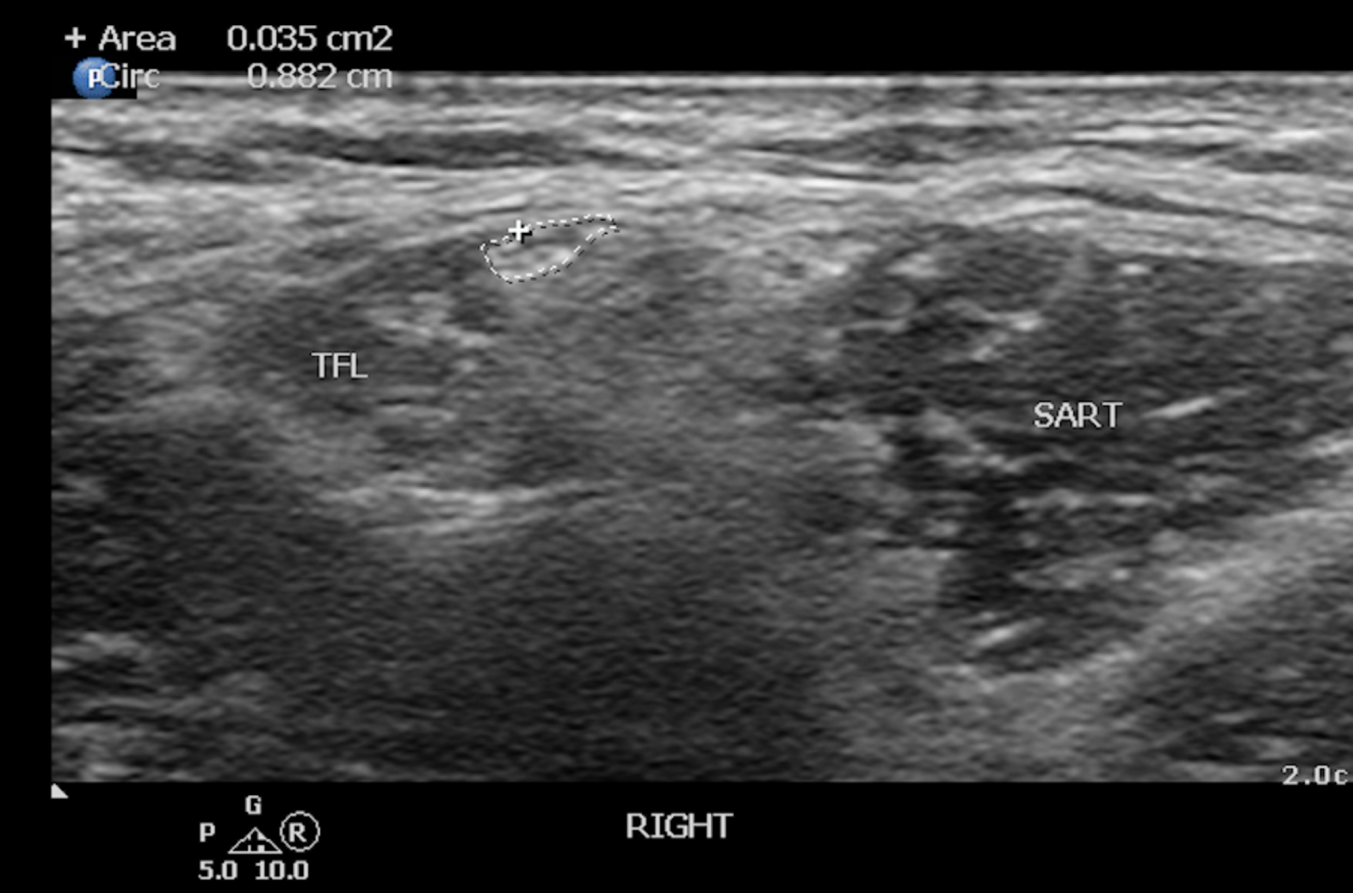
Ultrasound localization of the lateral femoral cutaneous nerve This ultrasound screen capture demonstrates the location of the right lateral femoral cutaneous nerve (circled) in the anterior thigh situated medial to the tensor fascia lata (TFL) and lateral to the sartorius (SART) muscle.
